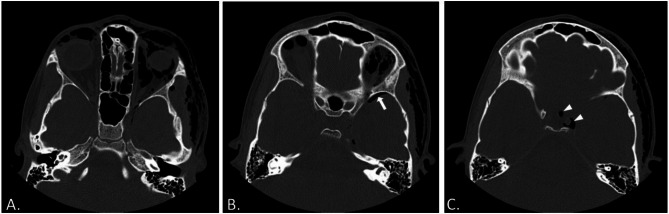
(A) Non-contrast axial CT of the brain and orbits demonstrating subcutaneous emphysema, bilateral orbital emphysema, with gas foci noted along the left superior orbital fissure. (B) Pneumocephalus observed in the left temporal lobe (arrow). (C) Cranial cuts showing gas foci along the left cavernous sinus and left parasellar area (arrow heads)
